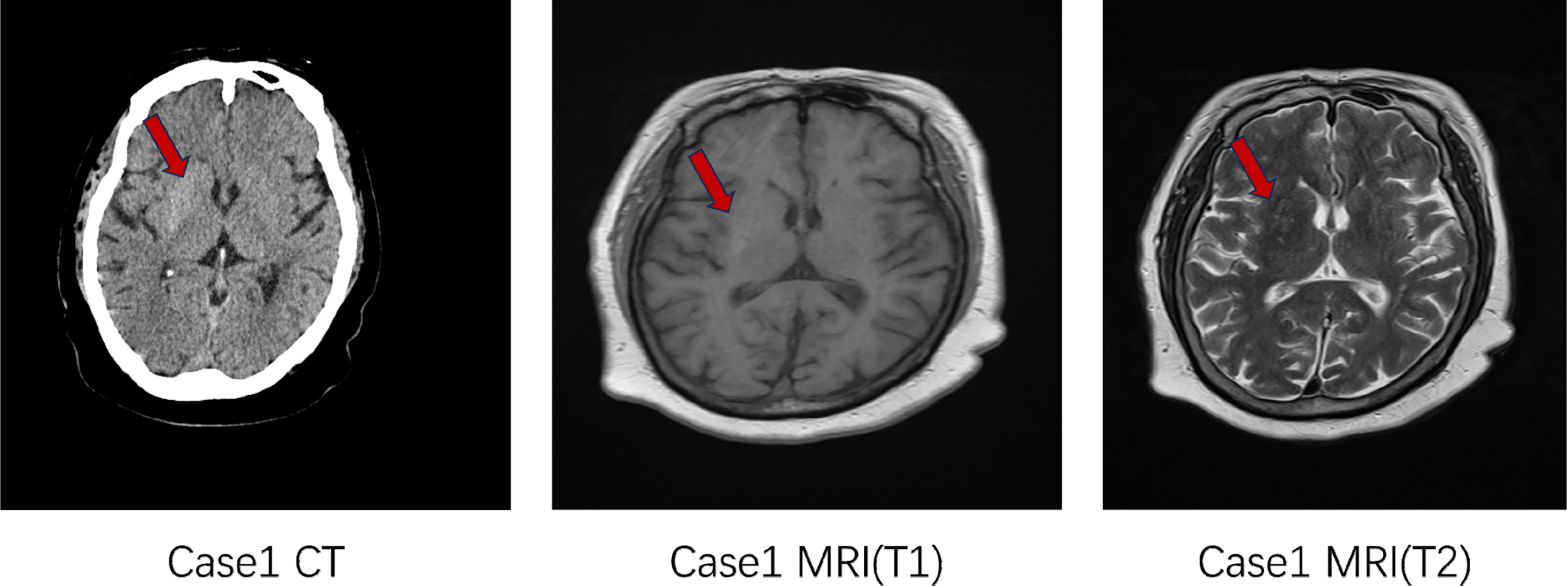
Imaging manifestation of case 1. CT, computed tomography; MRI, magnetic resonance imaging.
